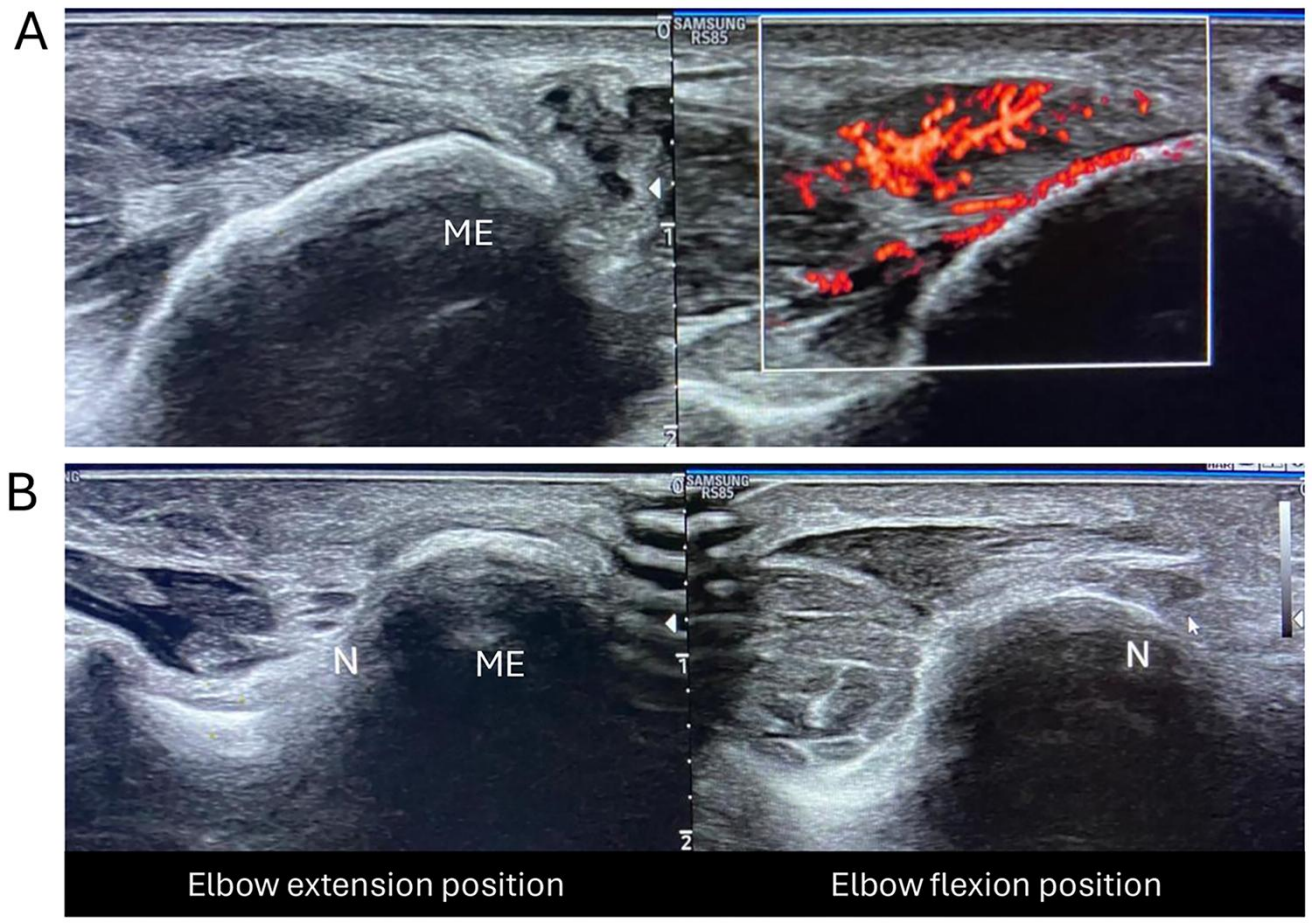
Ultrasound imaging of the medial elbow. (A) Longitudinal view and Color Doppler showing FPG common tendon entesopathy and tendinosis. (B) US axial view of ulnar nerve (N) displacement over the medial epicondyle (ME) during elbow flexion.
